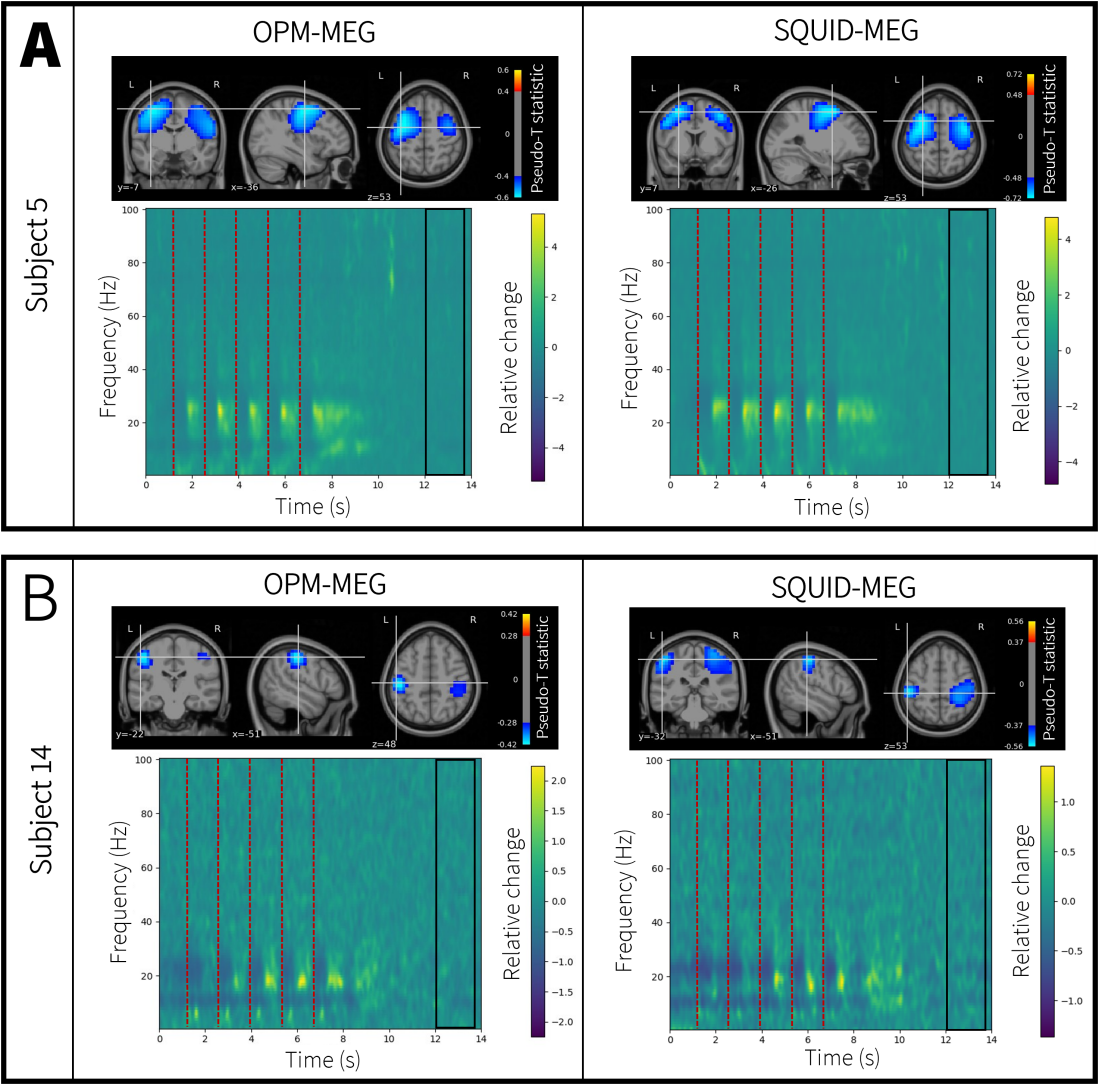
The basis of fingerprinting: Data from two representative subjects. The left panel shows OPM-MEG; the right panel shows SQUID-MEG. (A) Shows subject 5 and (B) shows subject 14. In all cases, the images show an MRI scan with the spatial signature of beta modulation (i.e., the pseudo-T-statistical image) overlaid in blue. The TFS from the left sensory cortex is also shown. Here blue represents a decrease in oscillatory amplitude relative to baseline. Yellow reflects an increase. Notice that whilst features are largely similar, there are differences between the two subjects that are maintained across the two scanner types.
